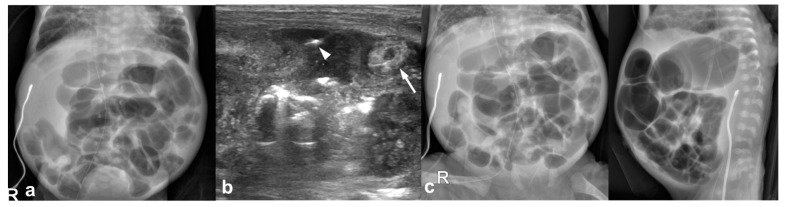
22-day-old girl (born at gestational age of 25 weeks and 3 days) with US-detected perforated necrotizing enterocolitis without radiographic pneumoperitoneum; this infant survived. (a) An abdominal radiograph obtained 7 h before US shows ileus with elongated loops and no pneumoperitoneum. (b) Preoperative US images show bowel wall thickening (arrow) and free air within the complicated ascites (arrowhead). (c) Preoperative abdominal radiographs obtained 30 min after US show no pneumoperitoneum.
